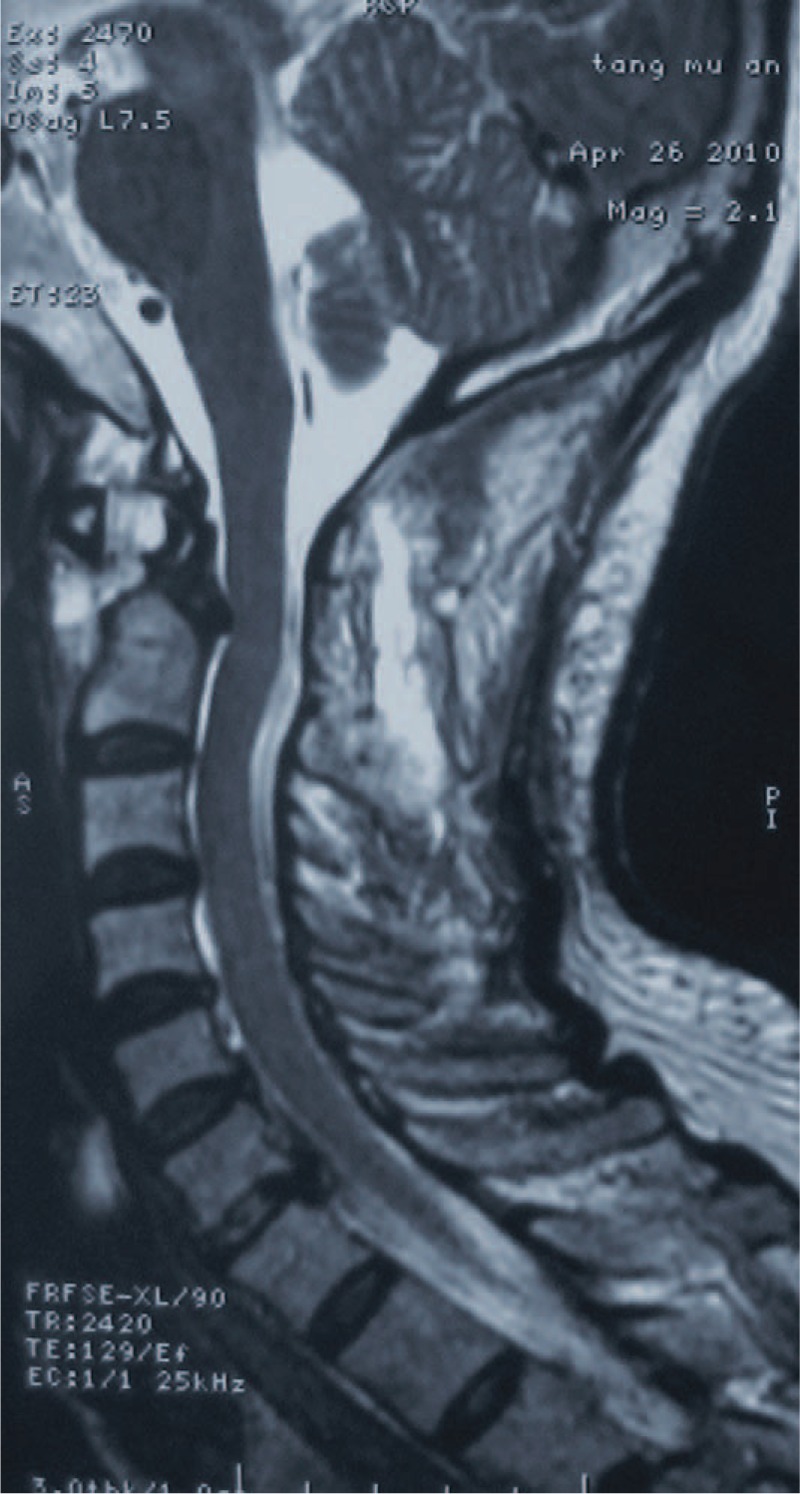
Postoperative sagittal MRI confirmed an anatomical reduction of the atlantoaxial complex and a normal spinal cord course without compression. MRI = magnetic resonance imaging.
